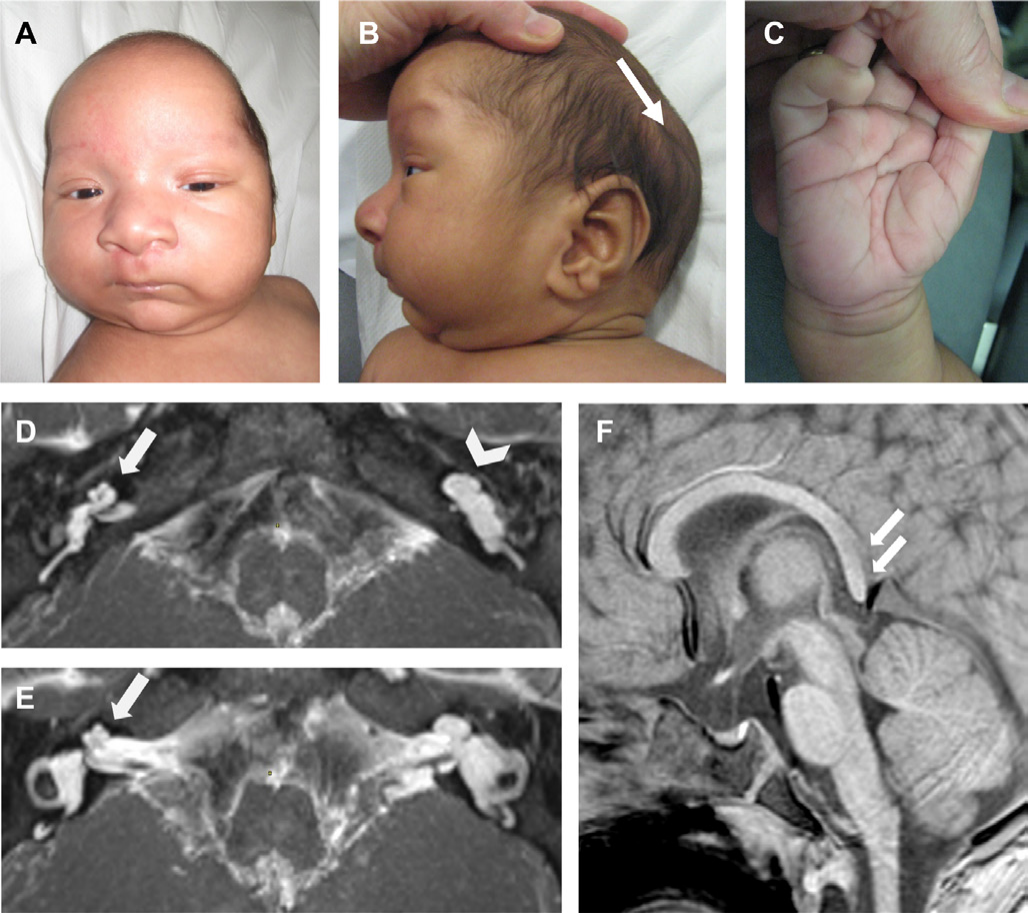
Clinical features of proband II-1 homozygous for *SPRY1* nonsense variant. (A–C) Photographs of the index patient aged 11 weeks showing scaphocephaly, turricephaly, bitemporal narrowing, hypertelorism, earlobe creases, cystic lesions overlying the lambdoid suture (B, arrow) and deep palmar crease (C). (D–F) Axial maximum intensity projection three-dimensional high-resolution T2-weighted images aged 8 months. (D–E) On his right side, he displays cochlear hypoplasia type 4 (arrows) with reasonably normal semi-circular canals. On the left, he has an incomplete partition type 1 cochlea (arrowhead), dilated vestibule with dysplastic lateral semi-circular canals. (F) Sagittal T1-weighted images showing small corpus callosum with hypoplastic splenium (double arrows).
